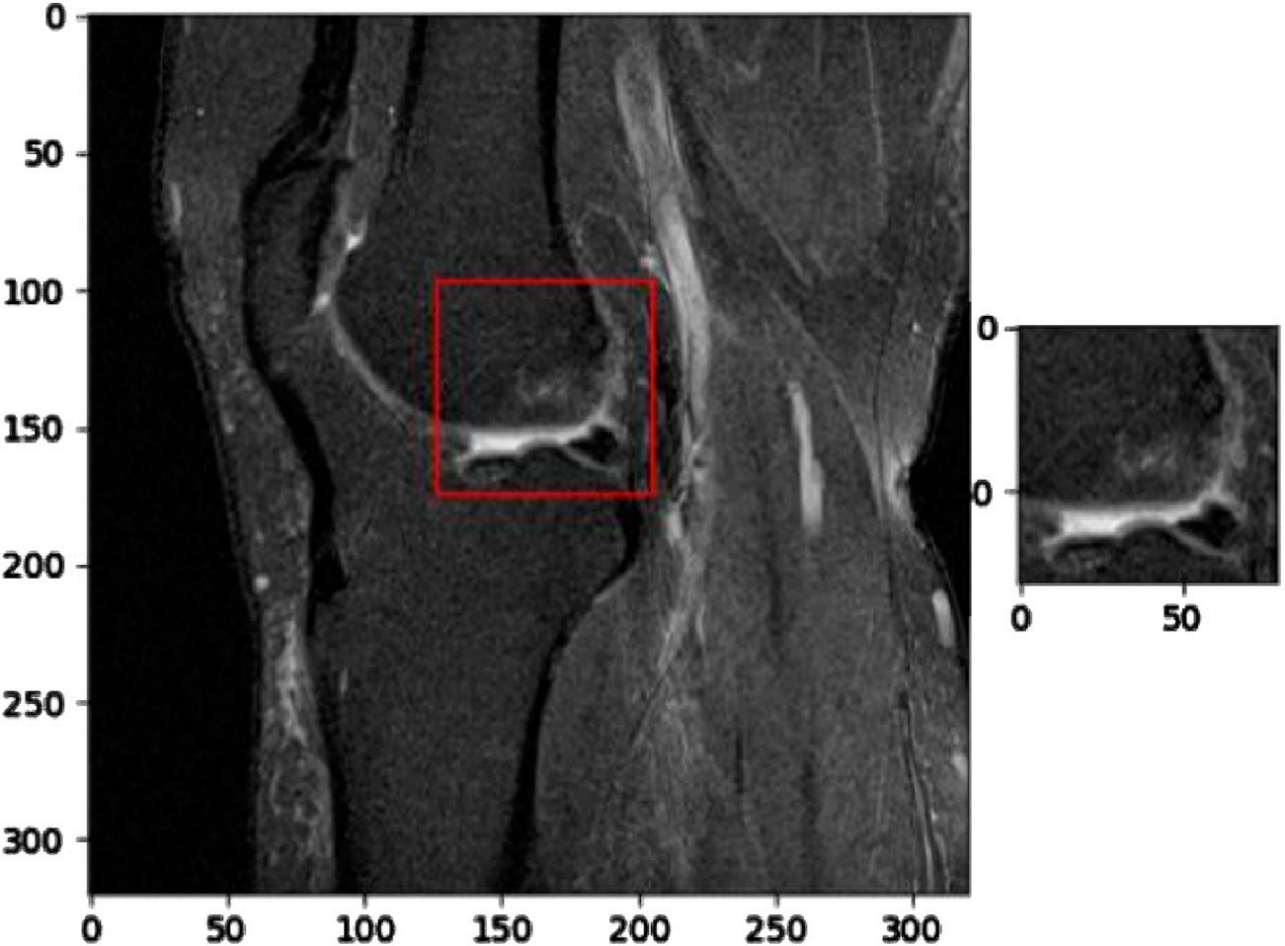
Segmented MRI image showcasing a partial anterior cruciate ligament (ACL) injury, demonstrating the ability of advanced image analysis methods to identify and localize even subtle signs of knee joint damage.
